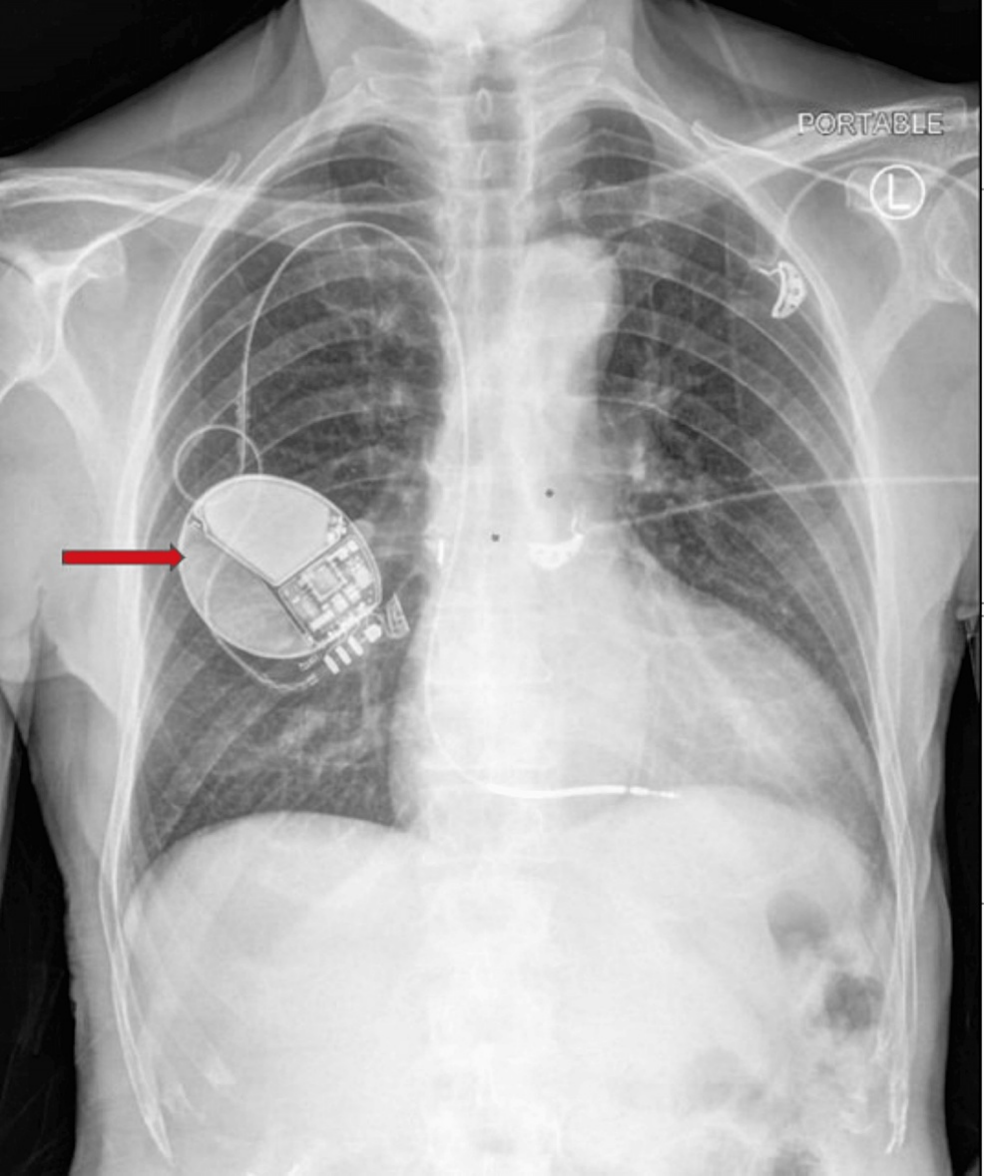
Initial chest X-ray demonstrating a right-sided automatic implantable cardioverter defibrillator (AICD).
